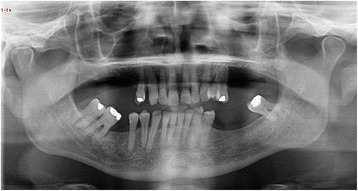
Panoramic dental radiograph showing dental status at presentation
